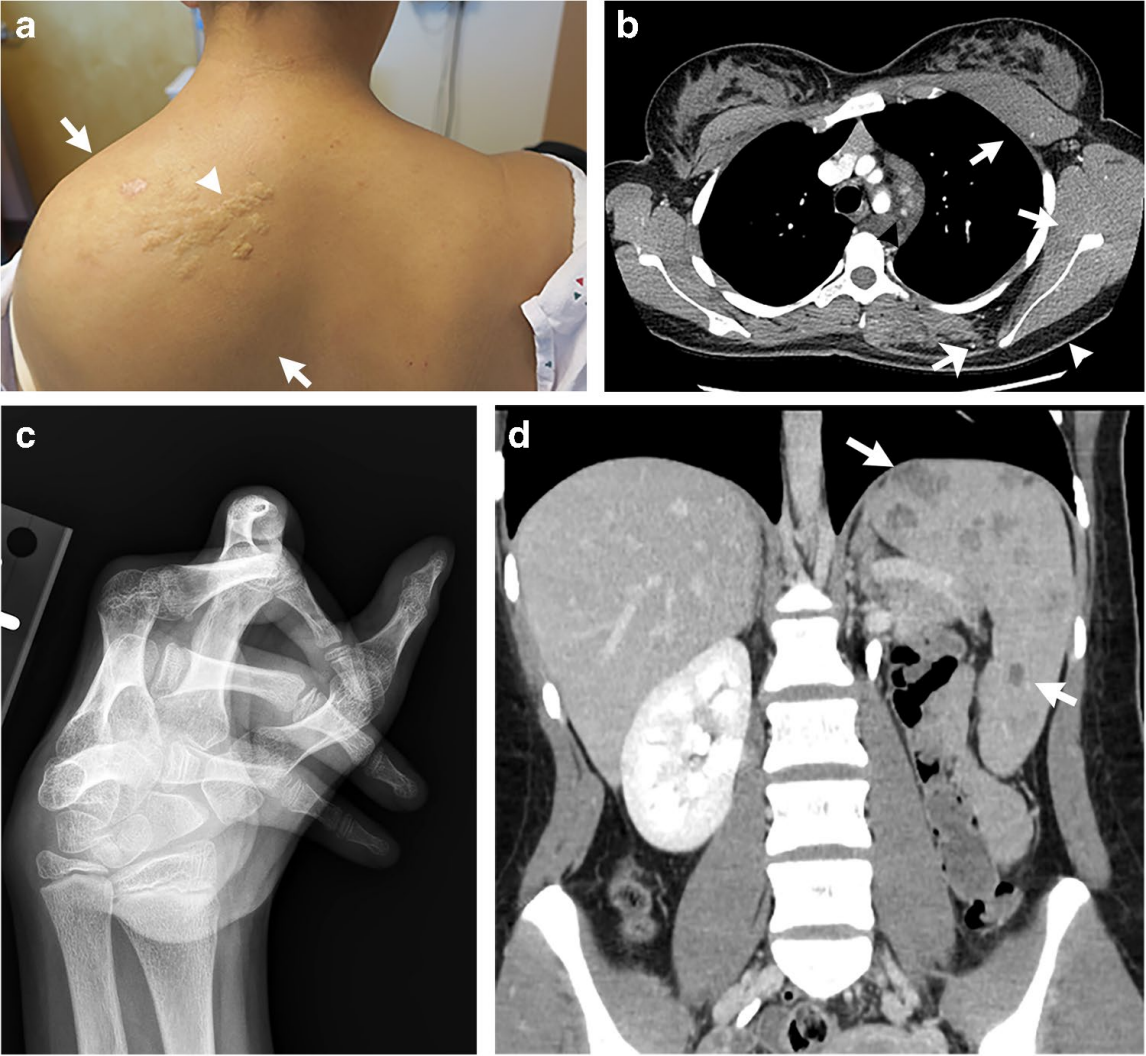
CLOVES syndrome in a 16-year-old girl with a genetically confirmed *PIK3CA* mutation with thoracic lipomatous overgrowth. a A clinical photograph shows left upper back overgrowth (*arrows*) that is typical of the CLOVES phenotype. Skin-colored papules (*arrowhead*) were also present that were consistent with collagenomas on biopsy. b An axial contrast-enhanced chest computed tomography (CT) shows enlargement of the left anterior and posterior chest wall muscles (*white arrows*) and subcutaneous fat (*white arrowhead*). Venous malformations are present in the left mediastinum (*black arrowhead*) as well as the left back with an associated phlebolith (*wide white arrow*). c A posteroanterior radiograph of the hand shows deformity diagnostic of arthrogryposis. d A coronal contrast-enhanced CT abdomen shows multiple splenic lesions (*arrows*) that also represent vascular malformations
